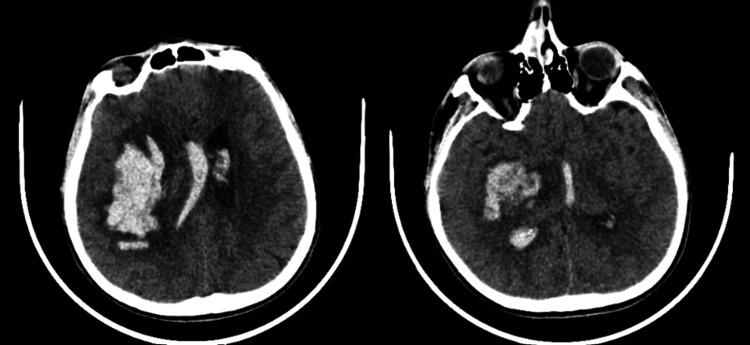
Brain computed tomography imaging showing the hematoma causing a shift of the midline and opening into the ventricular system
